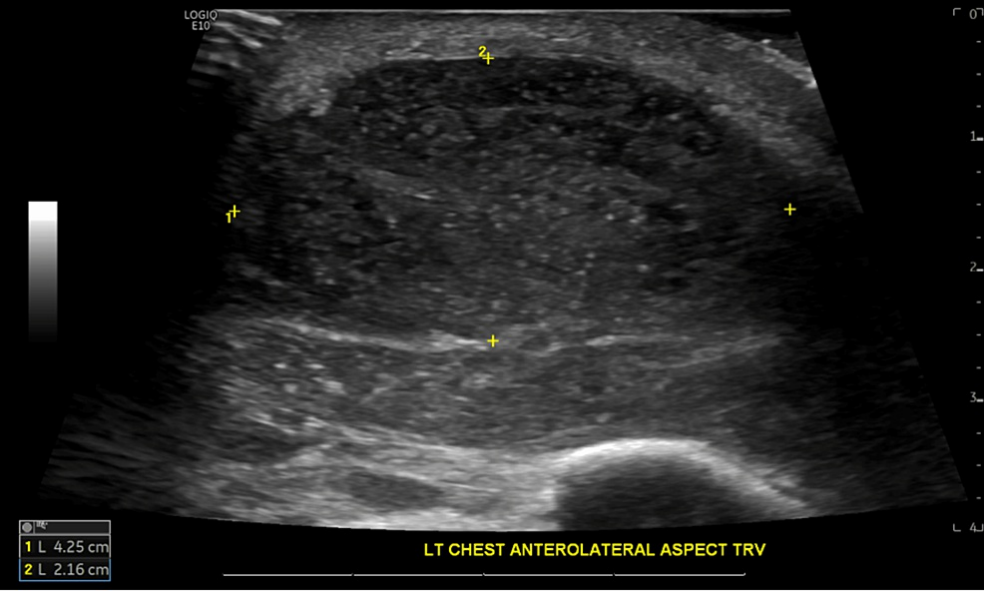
Chest ultrasound on day 1 of admission, showing a heterogeneous oval-shaped mass within the anterolateral aspect of the left chest wall.
